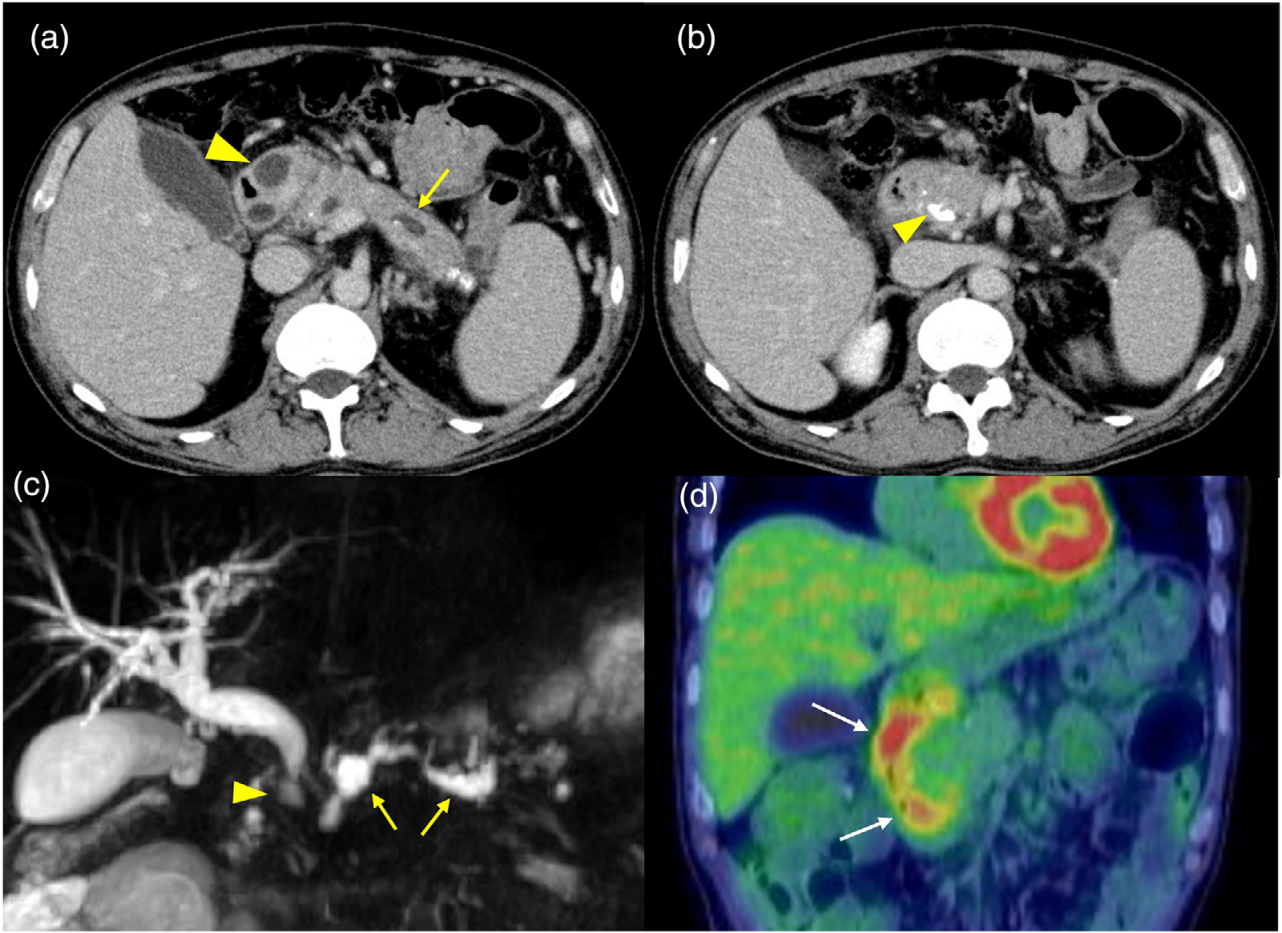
(a) Computed tomography: Duodenal wall thickening and cysts are observed (arrowhead). The main pancreatic duct is dilated (arrow). (b) Computed tomography: The pancreatic head is enlarged, and the surrounding fatty tissue density is elevated. A pancreatic stone is seen in the pancreatic head (arrowhead). (c) Magnetic resonance cholangiopancreatography: The distal bile duct is gently and smoothly stenosed (arrowhead), and the bile duct is dilated. The main pancreatic duct also shows stenosis at the head and cyst‐like dilatation toward the body's tail (arrow). (d) Fluorine‐18‐deoxyglucose: Fluorine‐18‐deoxyglucose was accumulated from the upper part of the duodenum to the inferior duodenal angle (arrow). The accumulation was along the wall of the duodenum and was considered due to inflammation in the groove area
